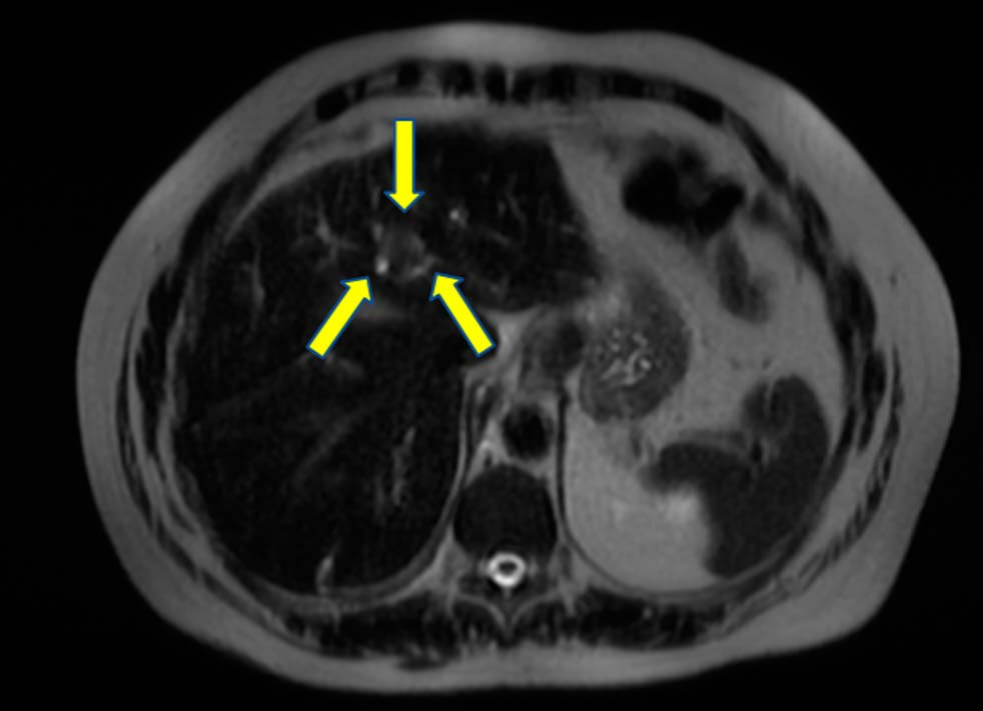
T2-weighted imaging of the liver mass.
